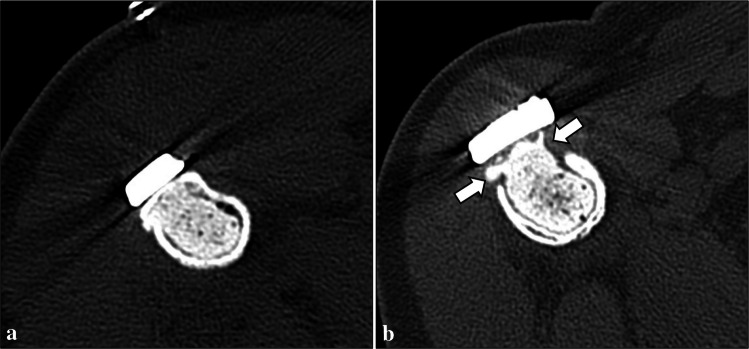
Cement loosening in a 51-year-old woman. After curettage of an ACT of the proximal humerus, the cavity was entirely filled with cement on the CT scan performed on the day of surgery (a). Cortical and cancellous bone resorption (arrows) progressively occurred around the cemented area after surgery, as shown on CT at 2-year follow-up (b)
